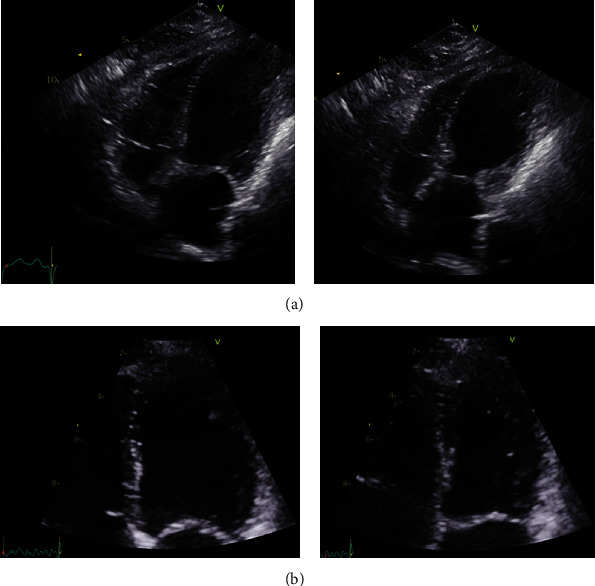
(a) Echocardiography in the acute phase. The first echocardiography showing modified 4 ch view of end-diastolic and end-systolic left ventricular volume. Basal hypercontraction and apical ballooning is evident, interpreted as acute heart failure/takotsubo cardiomyopathy. (b) Echocardiography after remission of tension pneumothorax and subcutaneous emphysema. Follow-up echocardiography showing 4 ch view of end-diastolic and end-systolic left ventricular volume 1 month later. Left ventricular ejection fraction (LVEF) has normalized.
